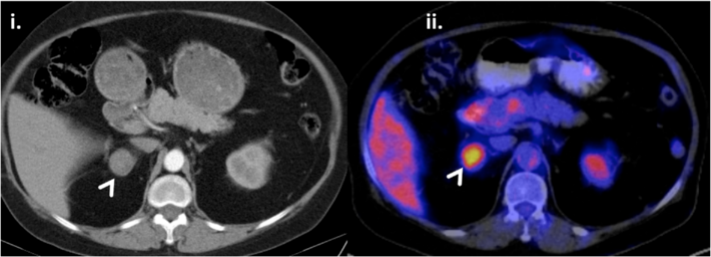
Imaging of the right adrenal tumor at initial evaluation. i. CT imaging revealed a 3.3 cm right adrenal tumor (white arrow) ii. High uptake of ^18^ F-FDG with standardized uptake value (SUV) of 5.0 for the right adrenal lesion (white arrow).
